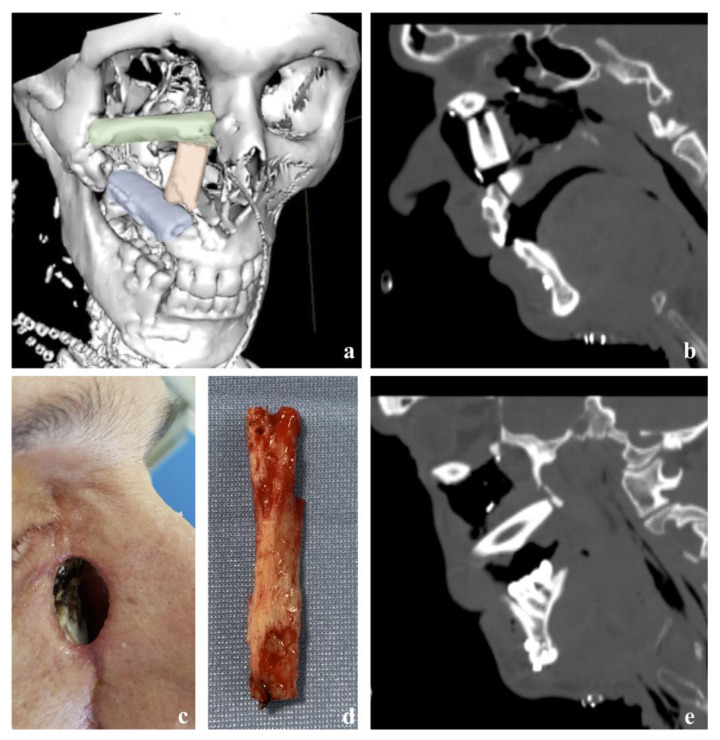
Partial flap failure of a free fibula flap used to reconstruct the left midface following the excision of a SCC originated from the maxillary sinus, with orbital invasion. (a) Three-dimensional view of the reconstruction. The pink segment, used to reconstruct the naso-maxillary buttress, was the one that subsequently had to be removed. (b) Post-operative CT showing the naso-maxillary segment. (c) Naso-cutaneous fistula determined by super-infection of the necrotic segment. (d) Naso-maxillary segment removed on post-operative day (POD) 23. (e) Post-operative CT showing the absence of the removed segment.
